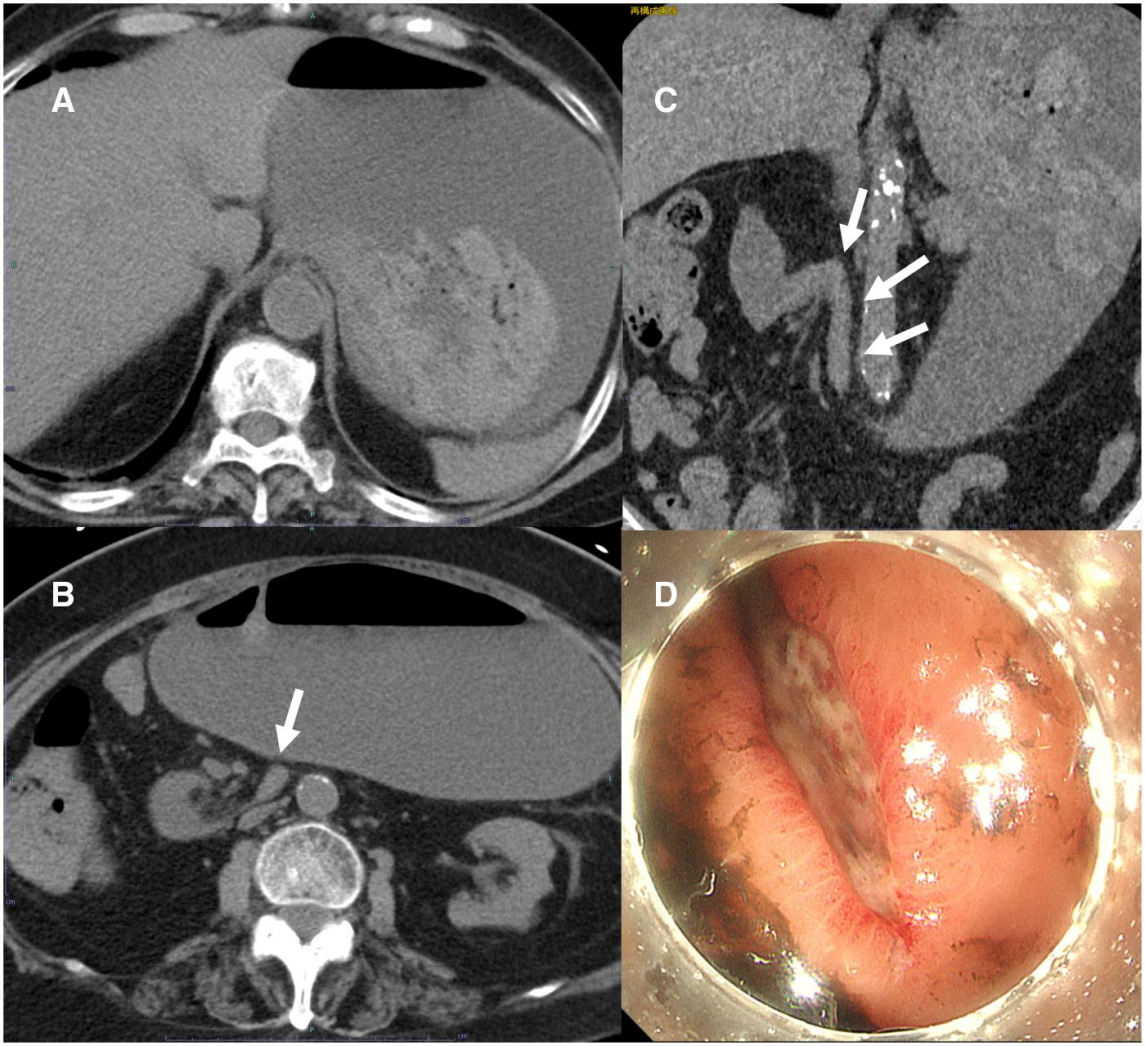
Axial non-contrast-enhanced CT images showing gastric dilatation and high-density contents that were considered a haematoma (A). Axial image (B) and coronal image (C) showing that the third part of the duodenum is flexed steeply on the right side of the aorta (arrow). Upper gastrointestinal endoscopy showing a laceration at the gastric cardia (D).
